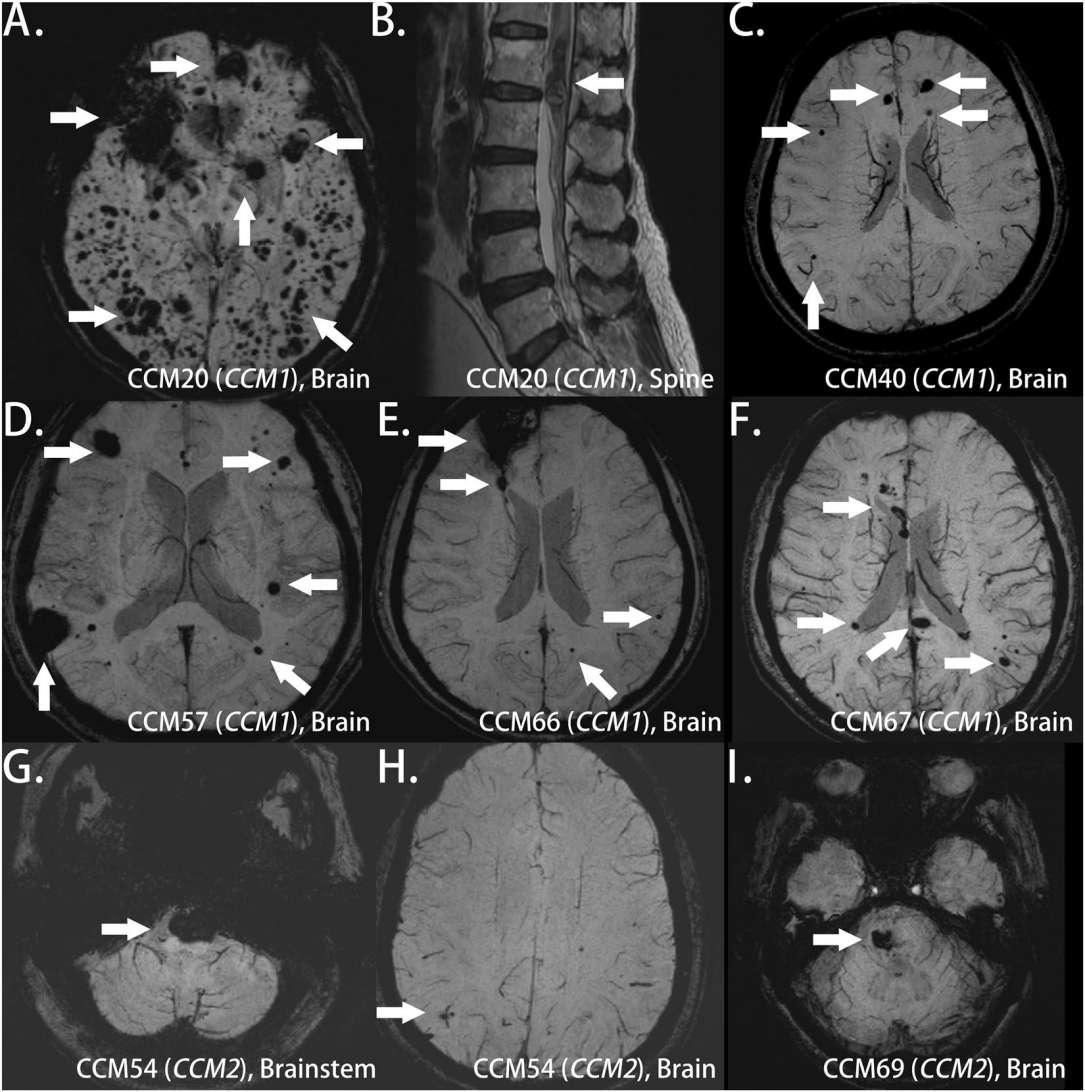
Brain MRI of patients with CCM variants. (A) Patient 20 with two *CCM1* variants: multiple lesions of variable sizes in hemispheres in susceptibility weighted imaging (SWI); (B) Patient 20 with one spinal cord lesion in a T2-weight image; (C) Patient 40 with one *CCM1* variant: multiple small lesions in hemispheres in SWI; (D–F) Patients 57 (D), 66 (E), and 67 (F) from one family with one *CCM1* variant: multiple hemisphere lesions with variable sizes in SWI; (G,H) Patient 54 with one *CCM2* variant: one prominent pontine lesion (G) and small lesions in bilateral hemispheres (H) in SWI; (I) Patient 69 with one *CCM2* variant of uncertain significance: one lesion in the right pons and the other in the right basal ganglion (not shown) (arrow indicates the lesion).
